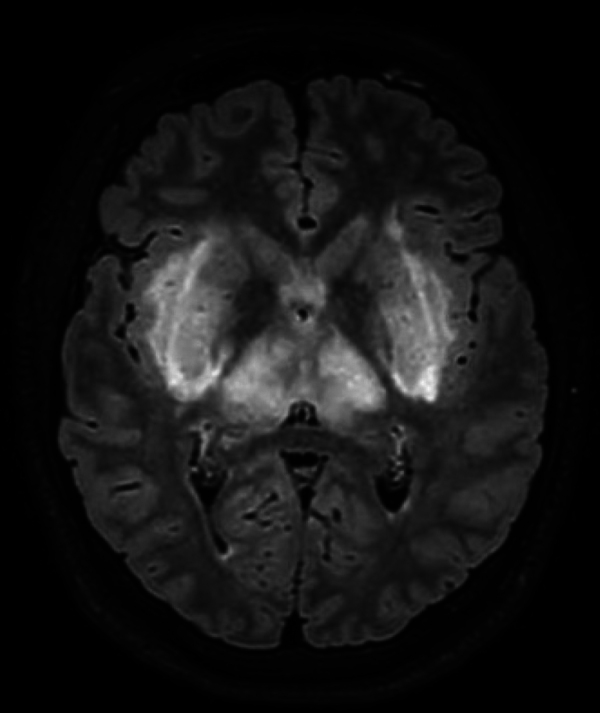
Axial FLAIR image showing hyperintensity in the lentiform nuclei and in the thalami.
